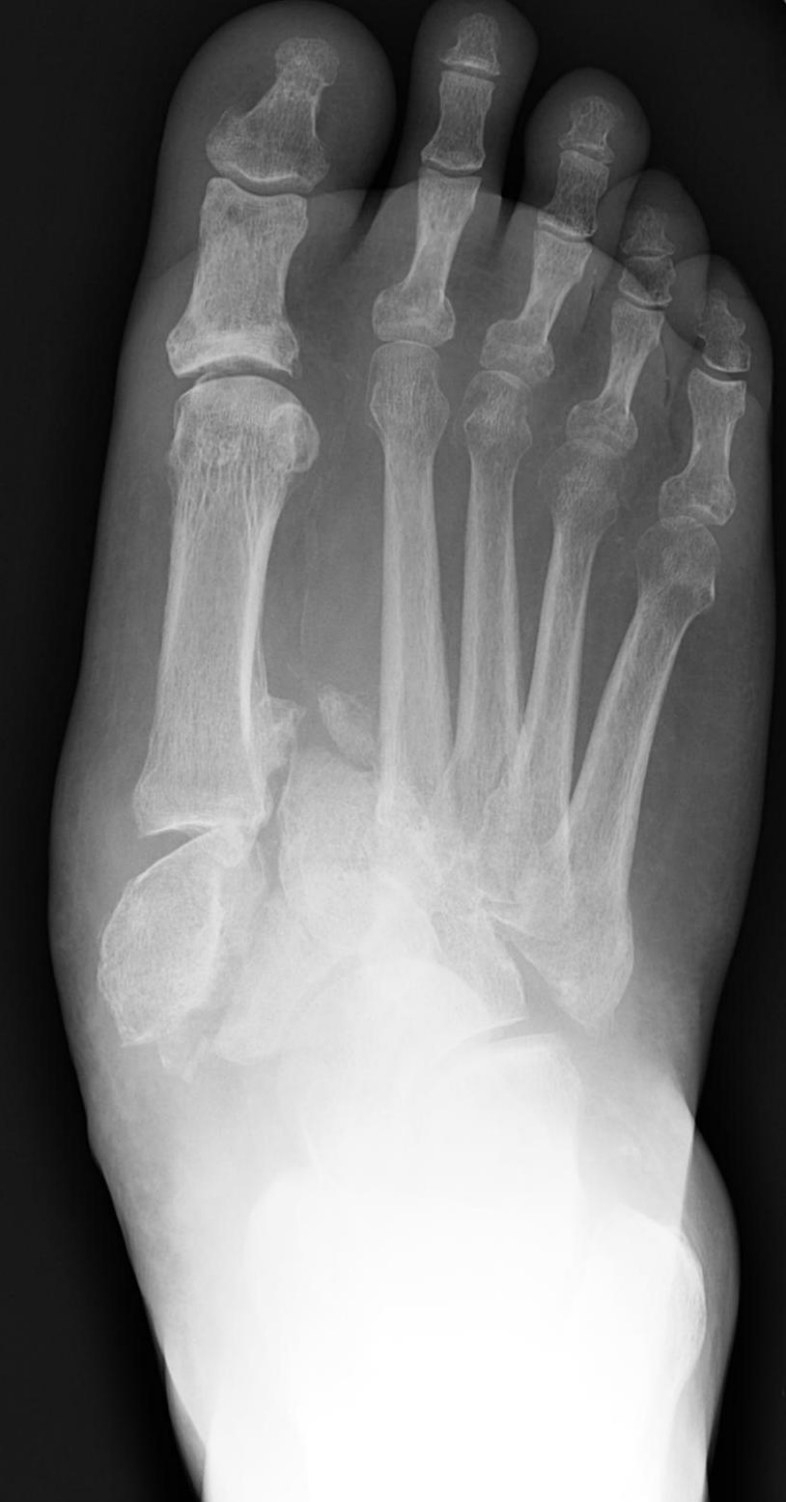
Preoperative anteroposterior radiographic view showing the severe midfoot fracture-dislocation of the diabetic CN foot.
